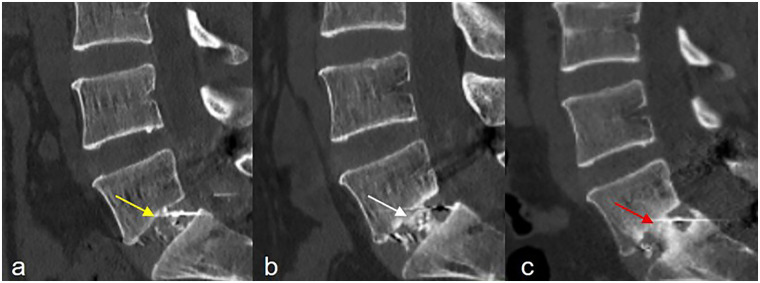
Grading definitions of the mBSF scale. (a) Grade I (Radiographic Pseudarthrosis): Presence of definitive signs of pseudarthrosis, such as a radiolucent zone surrounding the cage and the absence of bone bridge formation (Yellow arrow). (b) Grade II (Uncertain Fusion): Atypical radiographic findings where fusion status cannot be clearly determined; may be accompanied by partial callus formation or blurred borders (White arrow). (c) Grade III (Radiographically Stable Fusion): Demonstration of continuous trabecular bone bridging across the fusion space, with no radiolucent lines between the cage and endplates, indicating that solid bony fusion has been achieved (Red arrow).
